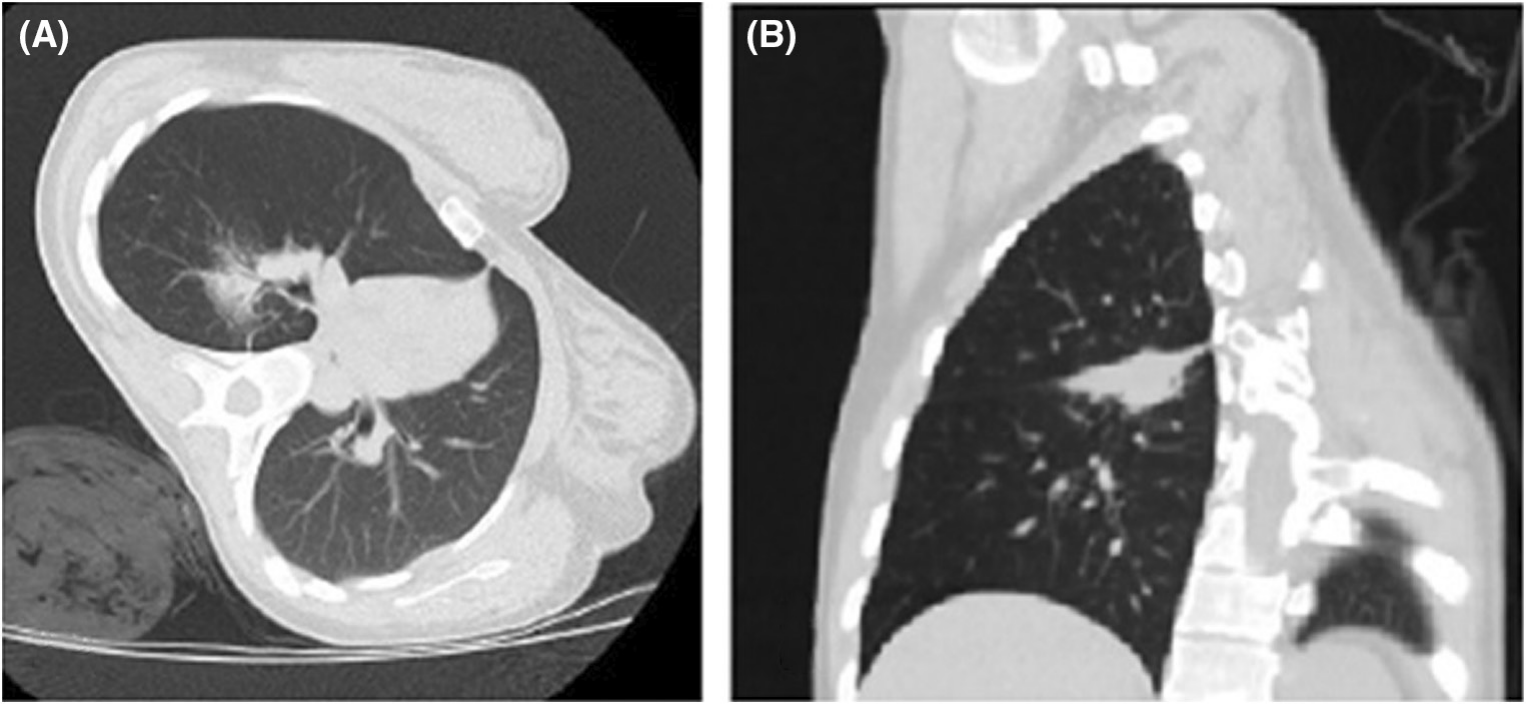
(A) Axial computed tomography view showing a lesion in the upper segment of the lower lobe on the right side. (B) Coronal computed tomography view.
